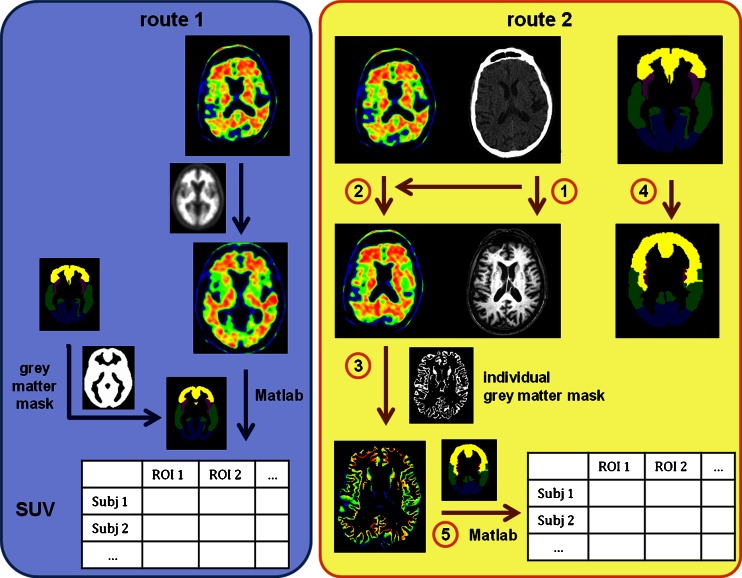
AV-45 quantification procedures: a cortical PET template-based (*route 1*) and b cortical MRI-based (*route 2*). a In route 1, AV-45 acquisitions were linearly registered onto a template from Avid (http://www.avidrp.com/), and regional uptake was quantified for each subject in the MNI space using the AAL atlas [24] that was masked with a grey matter probability map. Uptake values were collected using Matlab. b In route 2, for each subject, the CT scan acquired together with the AV-45 image was registered onto the MRI anatomical space defined by the T1 image of the subject concerned (*1*). The transformation matrix obtained was then applied to the AV-45 image of the subject so that the AV-45 image was in the T1 space (*2*). A binarized grey matter mask obtained from MRI segmentation was applied to the transformed AV-45 image (*3*). The AAL atlas was also registered onto each individual T1 space using the inverse of the transformation matrix from the T1 registration onto MNI space (*4*). Regional cortical uptake was collected for each subject using Matlab (*5*). *Arrows* represent transformation (*Subj* subject)
